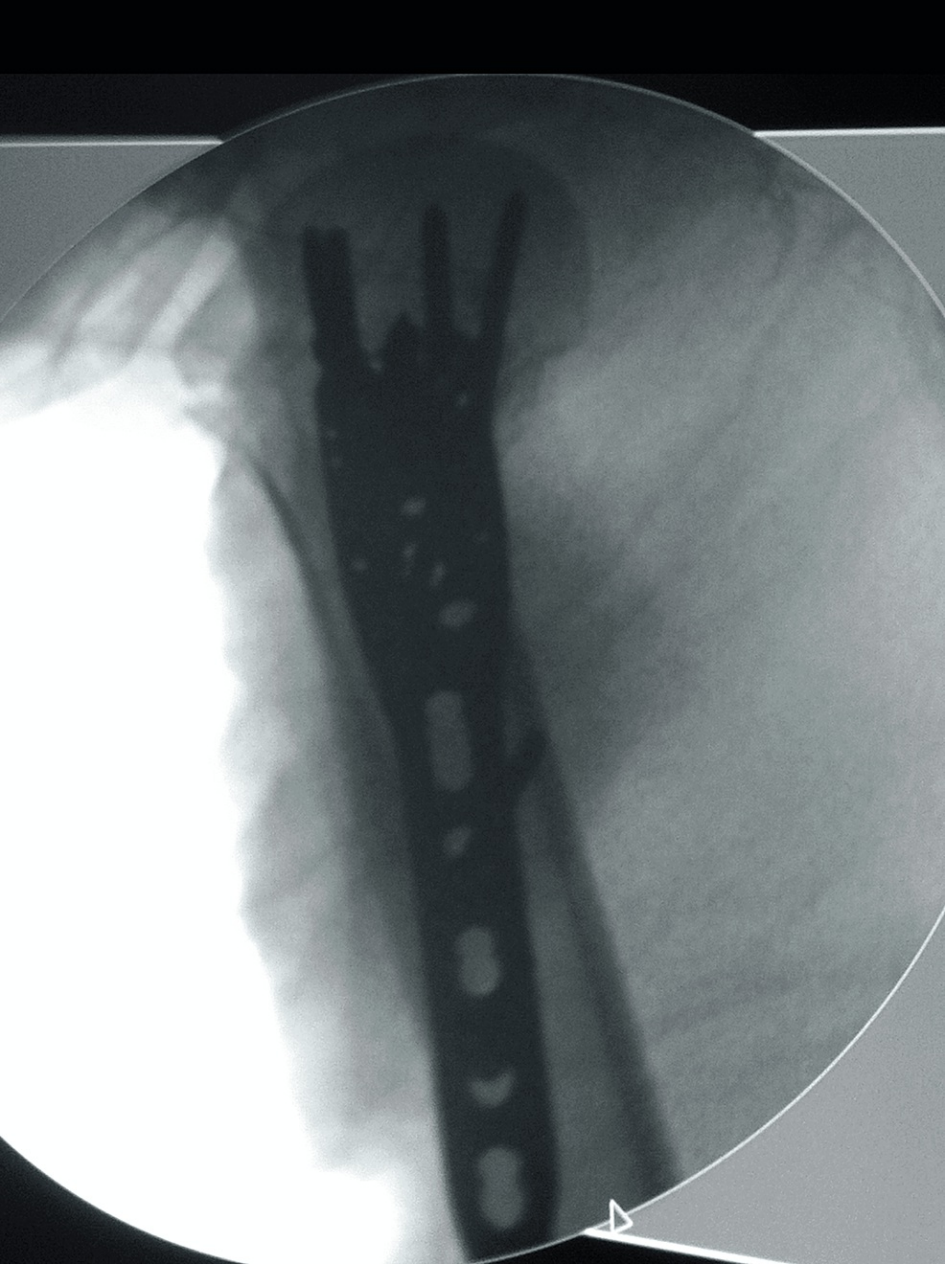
Intra-operative radiograph showing lateral view of applied PHILOS plate PHILOS: Proximal Humeral Interlocking System
